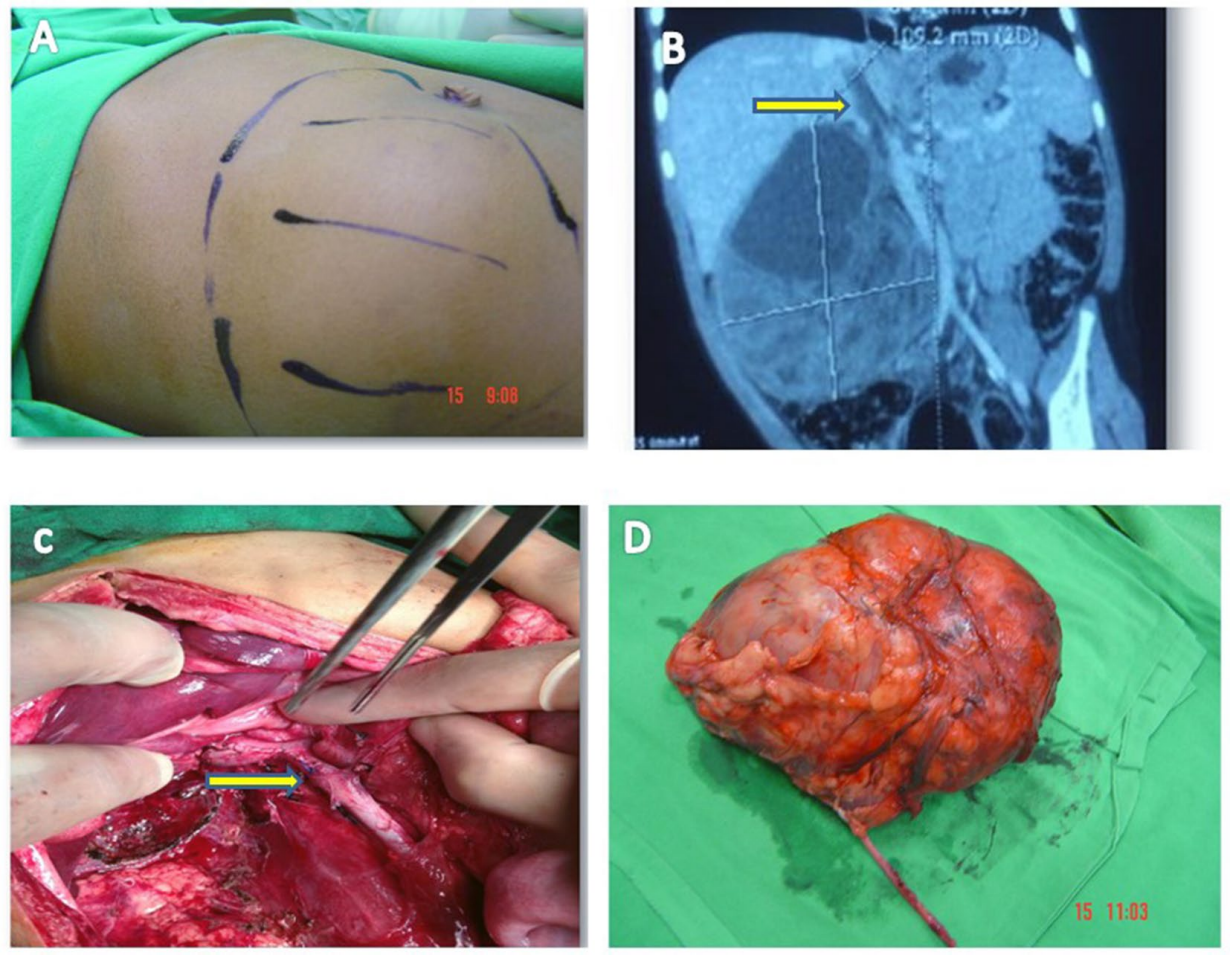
Photographs of a patient 3 years of age who presented with an asymptomatic abdominal mass noticed by mother including: (A) Preoperative picture with incision site marked; (B) representative coronal contrast-enhanced CT image of the same patient showing right-sided renal tumor along with a tumor thrombus extending into IVC (arrow); (C) representative intra-operative picture of the same patient where IVC was opened and thrombus retracted completely followed by repair of the vessel at the selected site (arrow); (D) Excised tumor mass
